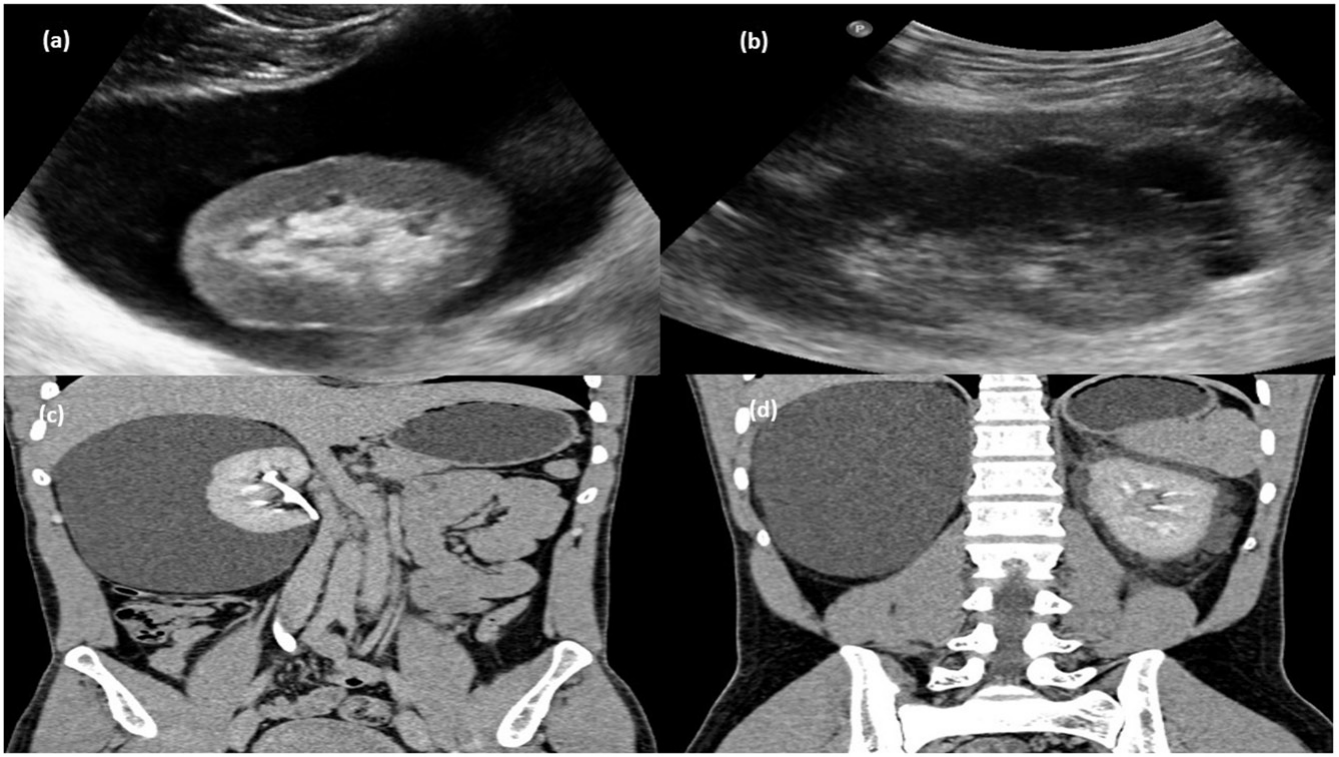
Gray-scale ultrasound image (a) of right kidney showing anechoiec collection in perinephric region and (b) left kidney showing multi septated anechoic collection in perinephric region. (c) CT scan arterial phase in coronal plane showing nonenhancing collection (d) MPR reformation of coronal plane of delayed phase of CT scan abdomen showing normal excretion of contrast.
